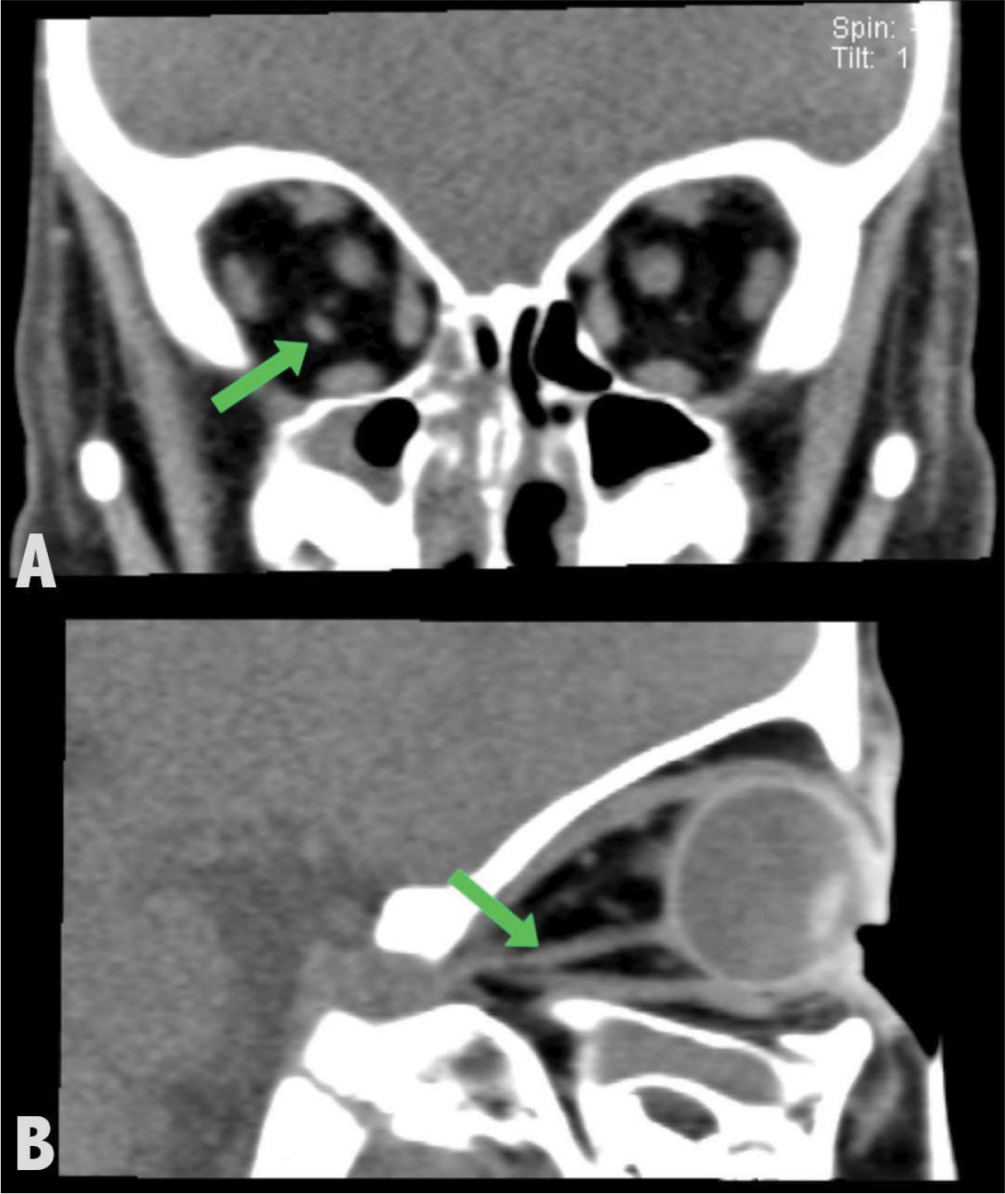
Unenhanced coronal (a) and sagittal (b) CT images of the orbits reveal a right accessory extraocular muscle (green arrows) located inferolateral to the optic nerve, medial to the lateral rectus muscle, and superior to the inferior rectus muscle. The muscle exhibits a density similar to that of the other extraocular muscles.
